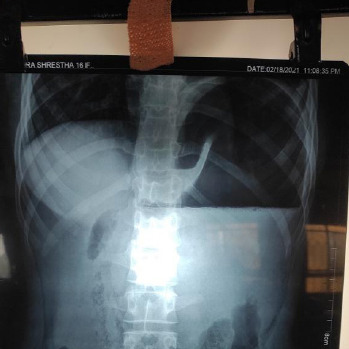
Abdominal X-ray of the patients revealed distended stomach with air fluid level.
